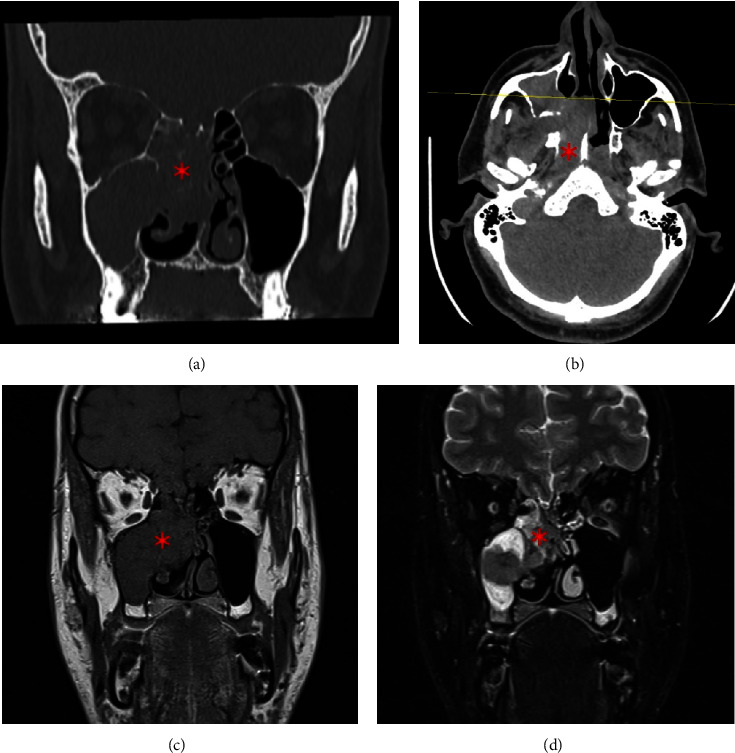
Computed tomography scans (upper left and right) and magnetic resonance imaging T1 (lower left) and T2 (lower right) of the destructive mass (red asterisk) in the right sphenoid sinus extending to different sinuses and the infratemporal fossa.
